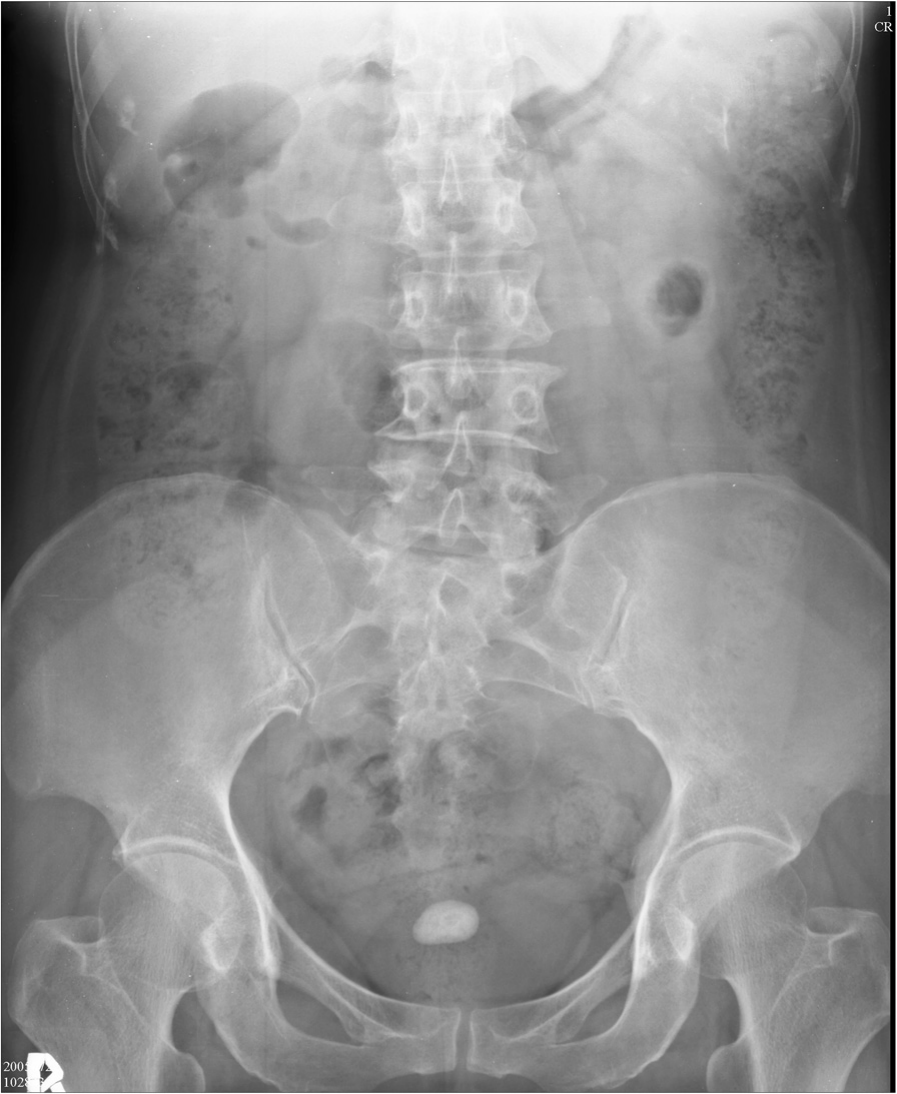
Abdominal X-ray showing a radiopaque shadow of 2.7cm × 1.5cm over the pelvis.
